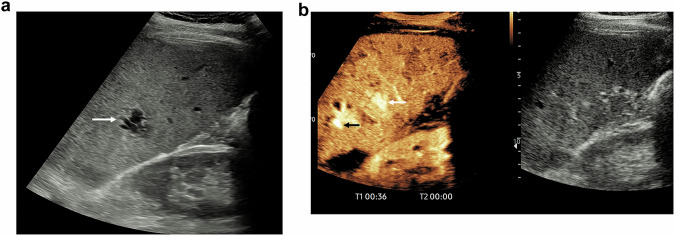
A 39-year-old male patient with BAT from a motor-bike accident, imaged by a targeted ultrasound examination to the site of small splenic laceration injury seen on the admission CT examination, day 5 post injury. a The B-mode ultrasound examination demonstrates a focal 12 mm low reflective irregular abnormality (arrow) without clear demarcation of a splenic laceration. b Following the administration of 1.2 mL of SonoVue™ (Bracco SpA, Milan), during the late arterial phase at 36 s, two pseudoaneurysms are present, the larger (white arrow) was seen on the B-mode examination, whilst the other (black arrow) was not seen. Further follow-up ultrasound examinations demonstrated resolution of the pseudoaneurysms
